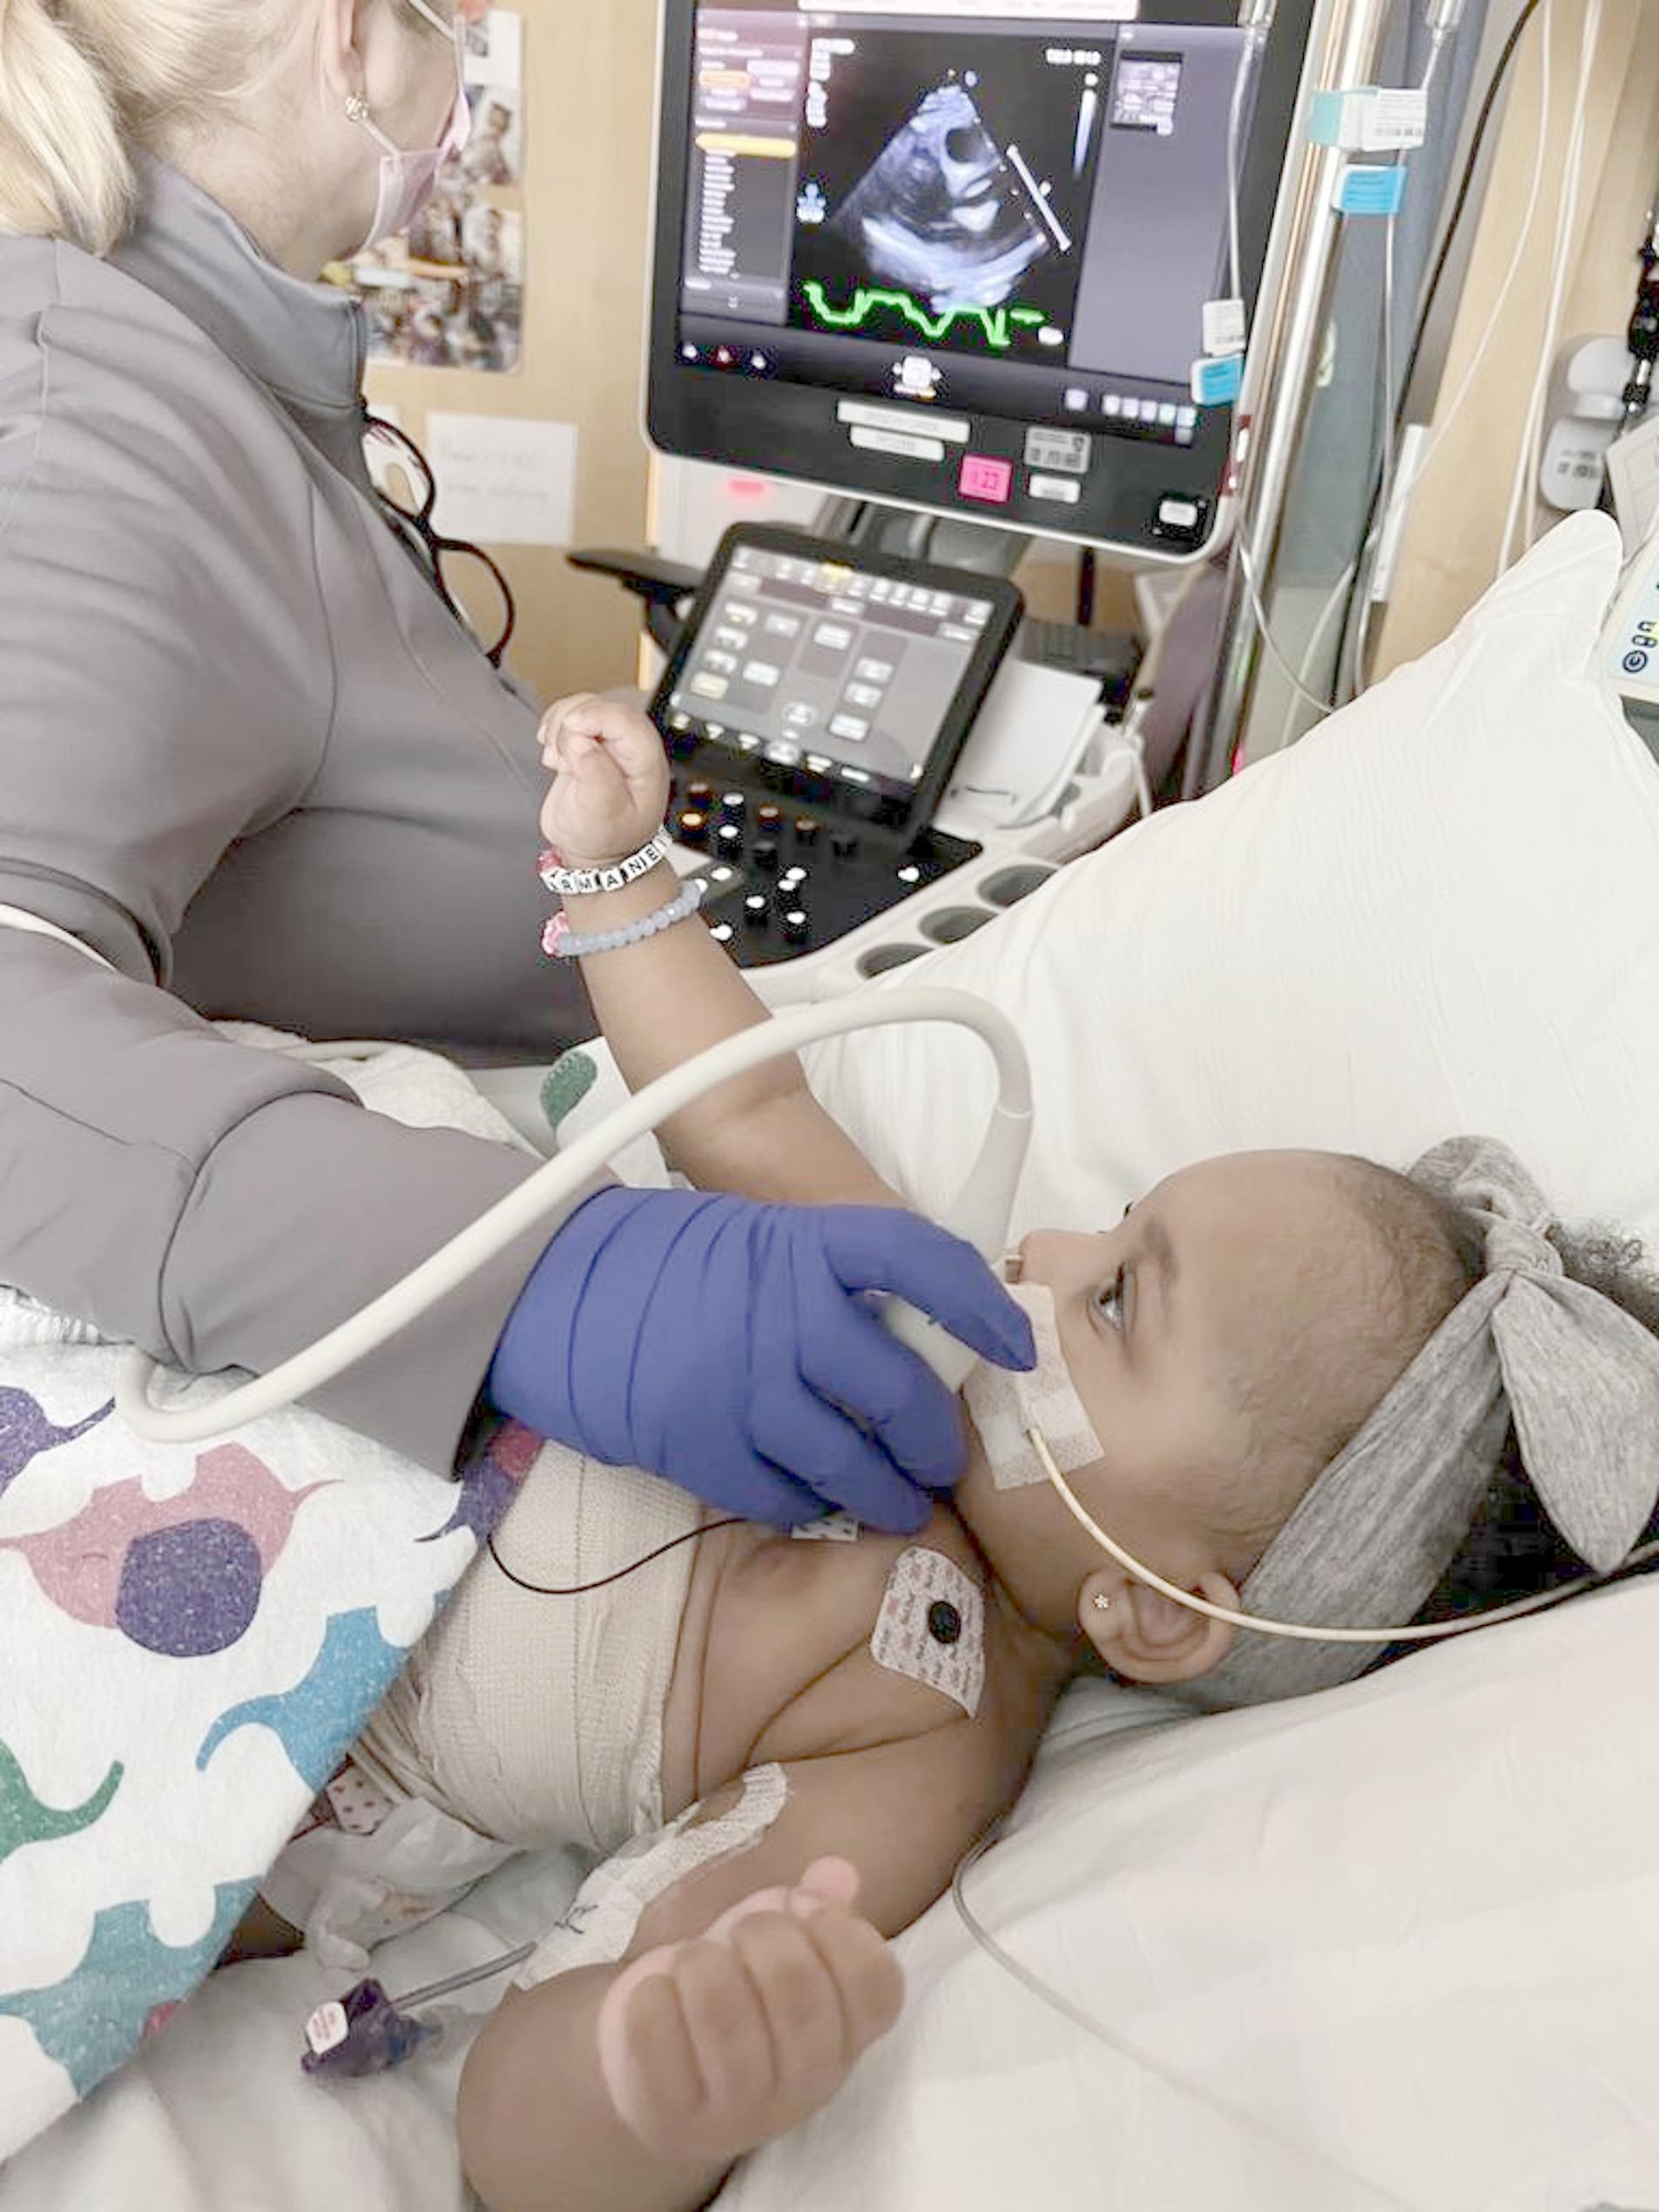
Photograph by adult caregiver of child getting echocardiogram
